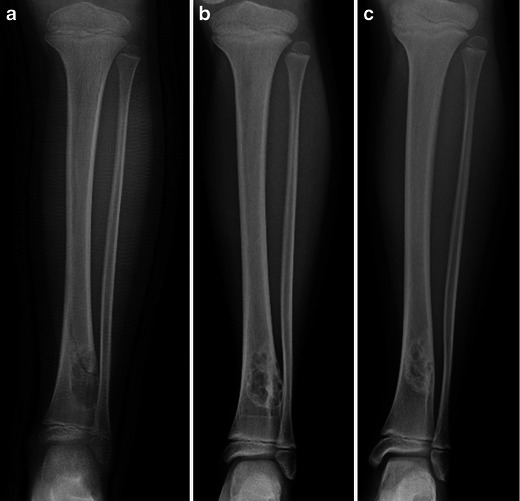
Frontal X-rays of the left tibia: Non-ossifying fibroma of the distal tibial metaphysis—evolution after fracture [age 7 (a), age 9 (b), age 11 (c)]
